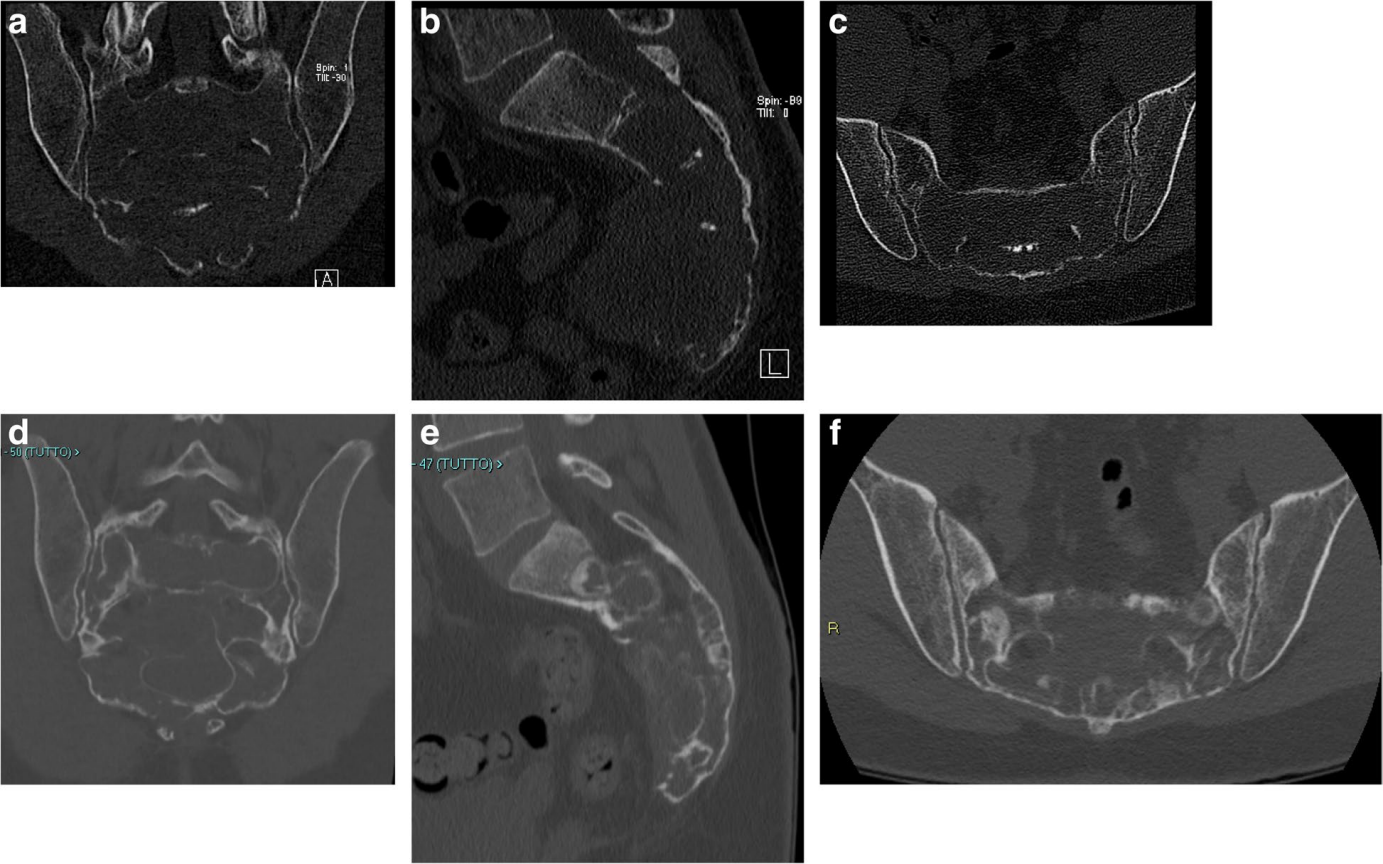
A case of sacral giant cell tumor of bone treated with no surgical treatment (denosumab alone) (Case 11). Computed tomography at presentation showed an osteolytic lesion of the sacrum (a: coronal view, b: sagittal view, c: axial view). Computed tomography showed bone sclerosis 3 years after the diagnosis, after 40 doses of denosumab treatment (d: coronal view, e: sagittal view, f: axial view)
